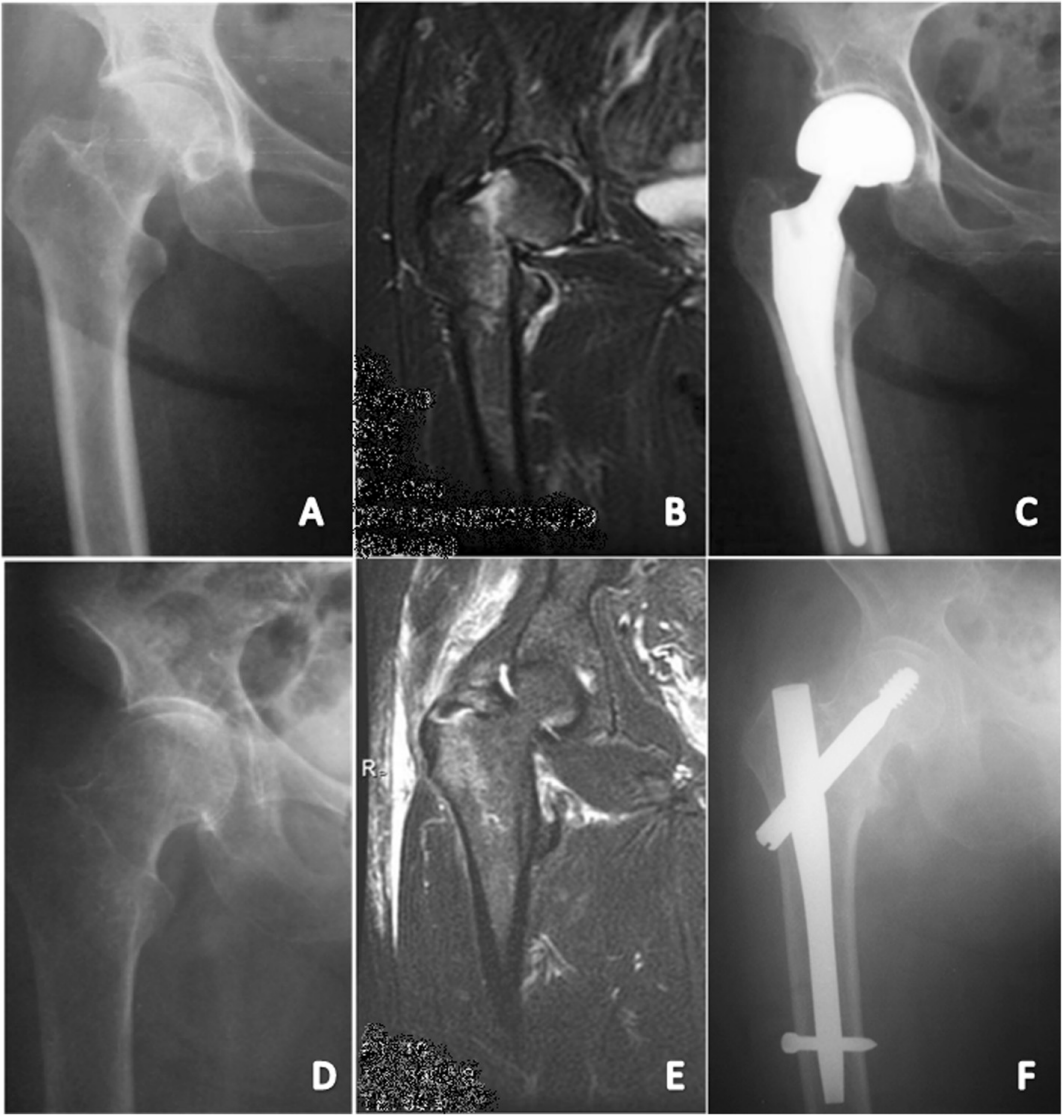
a X-Ray of occult femoral neck fracture of the right hip. b MRI of the same case showing a Garden II femoral neck fracture. c Postoperative X-ray showing a uncemented bipolar hip. d X-Ray of occult pertrochanteric fracture of the right hip. e MRI of the same case showing a incomplete pertrochanteric fracture (>50 %). f Postoperative X-ray showing osteosynthesis with a Gamma 3 nail (Stryker, NJ)
